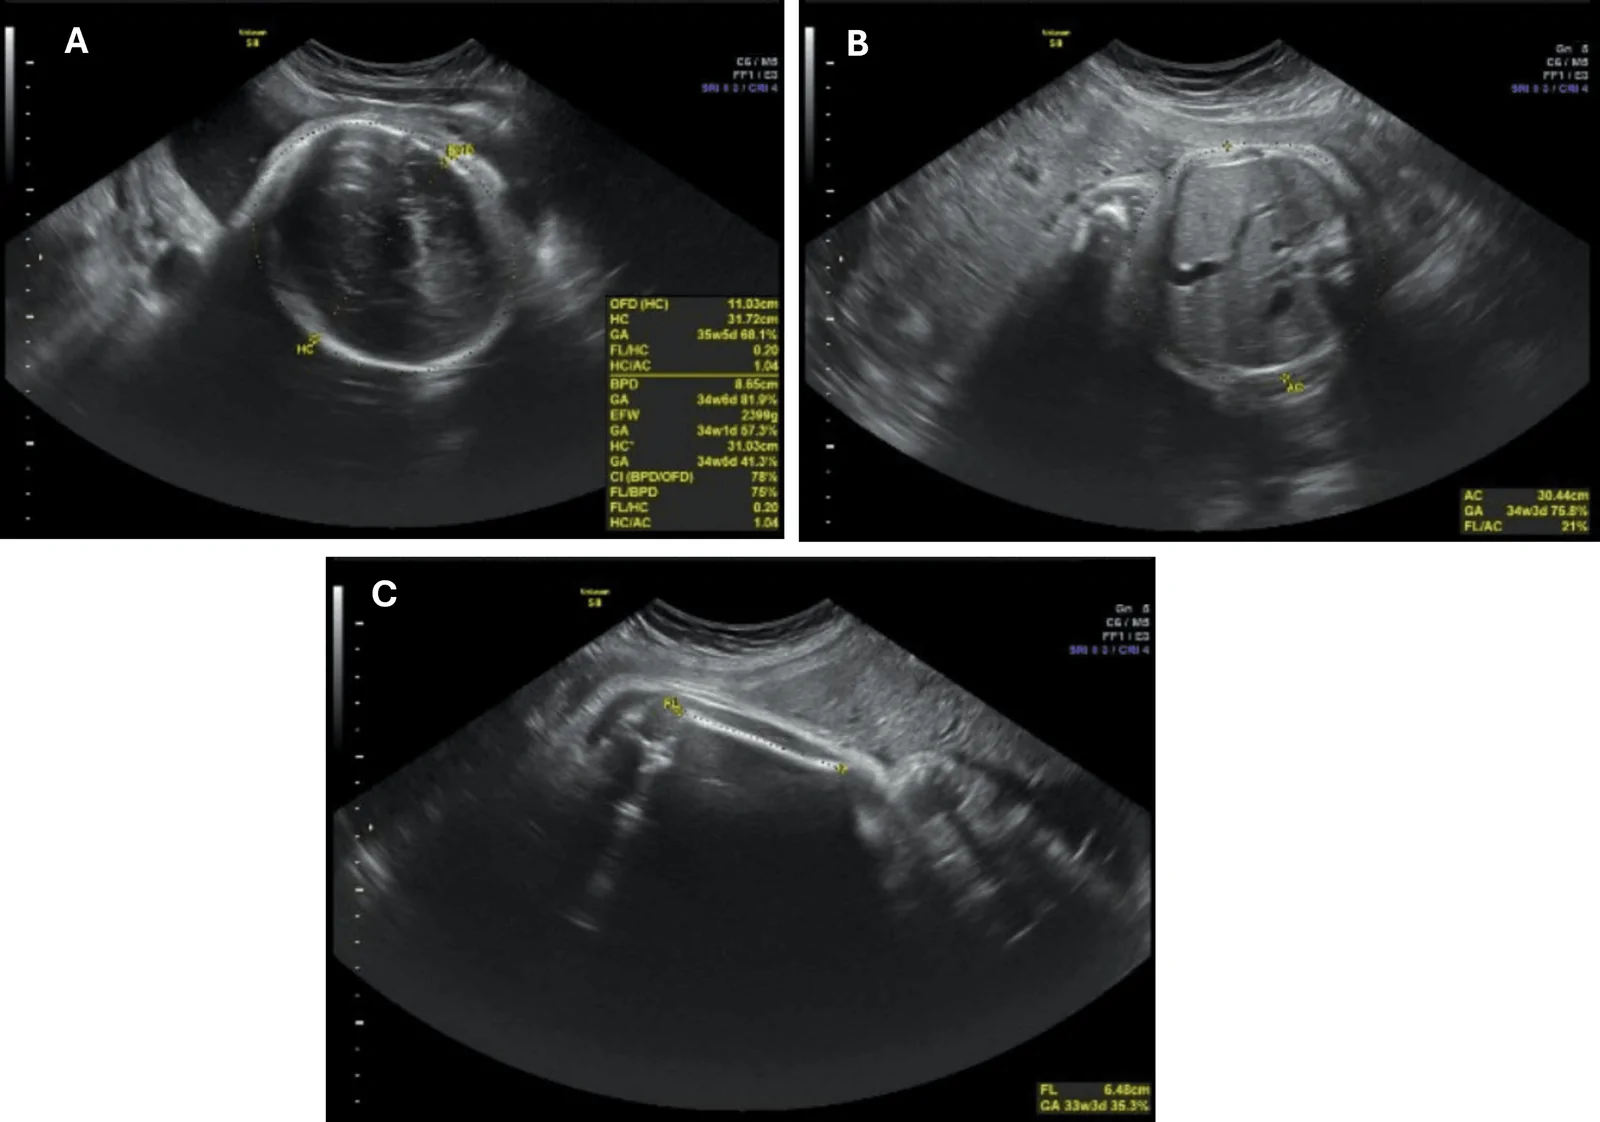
Obstetric ultrasonography demonstrating normal fetal growth parameters at 33 weeks' gestation (A) Measurement of the fetal biparietal diameter (BPD) and head circumference (HC). (B) Measurement of the fetal abdominal circumference (AC). (C) Measurement of the femur length (FL). All fetal biometric parameters were appropriate for gestational age, with no sonographic evidence of fetal growth restriction.
